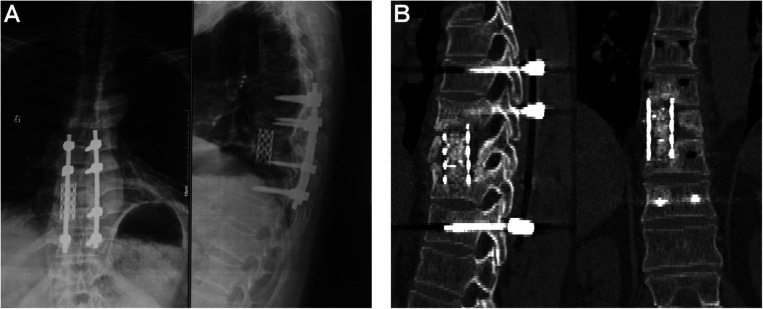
The radiographic and computed tomography images at the 2-year follow-up. (A) anteroposterior and lateral radiography; (B) CT images.
